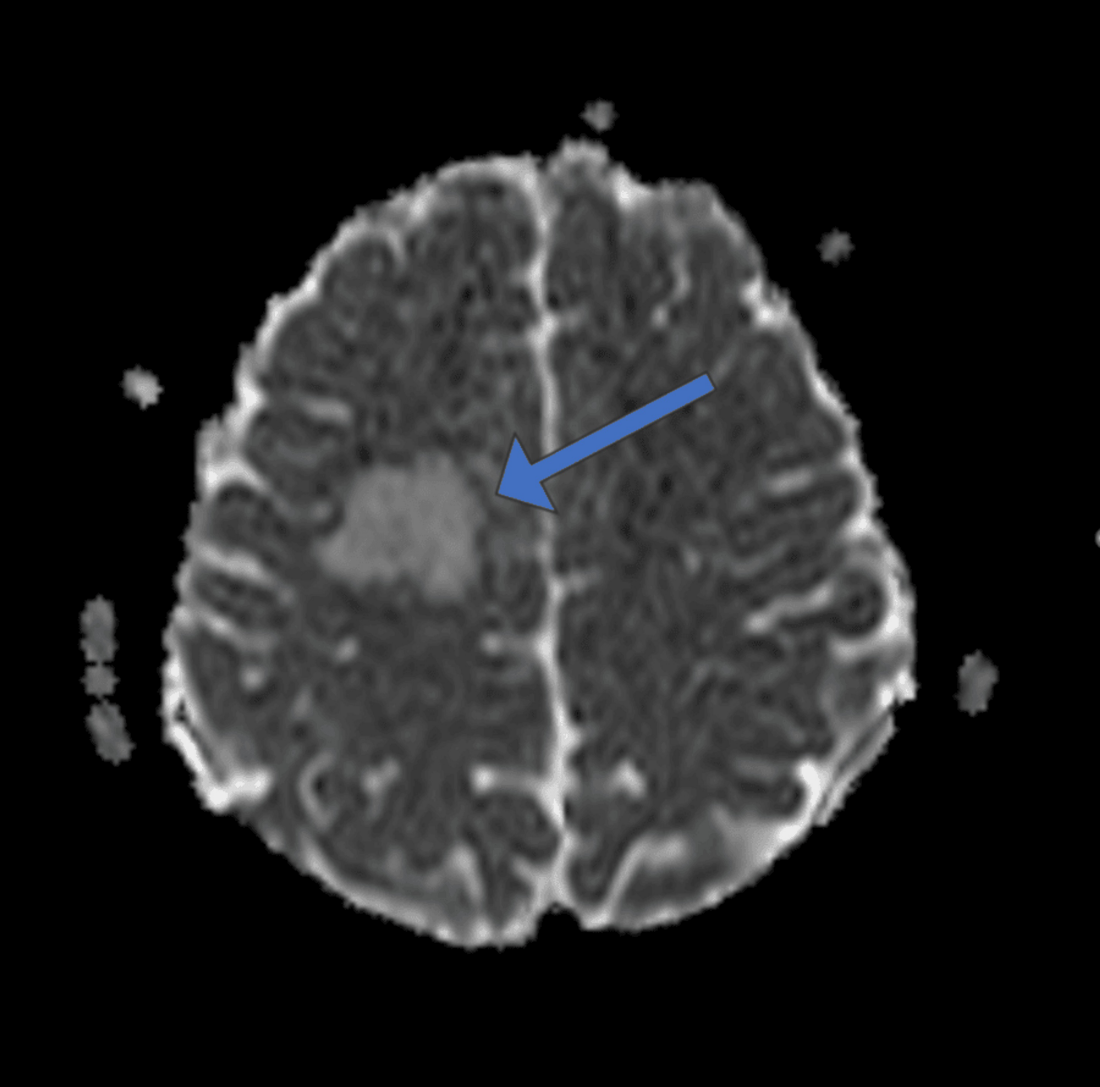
Axial ADC MRI image showing higher ADC values with corresponding hyperintensity at the lesion site in the right high frontal lobe (arrow). ADC, apparent diffusion coefficient
